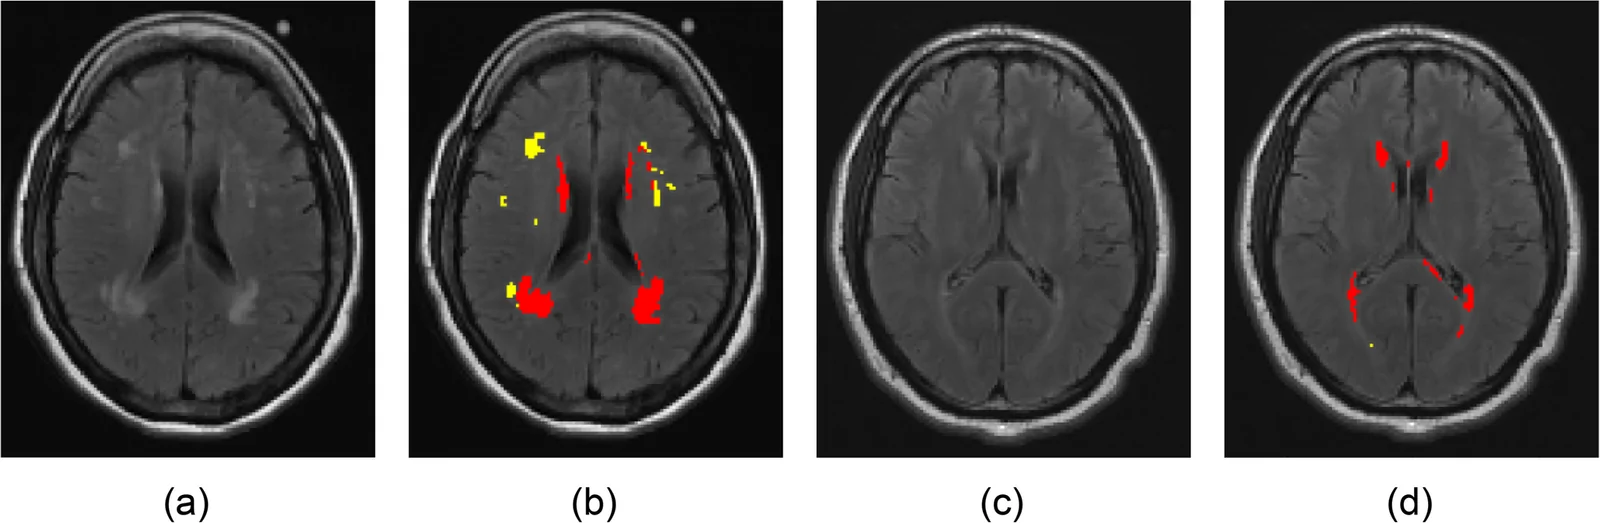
Axial FLAIR images and corresponding segmented WMHs using UBO detector from a representative HIV-only (a, b) and CU-only participant (c, d). pvWMHs and dWMHs are shown in red and yellow colors respectively
